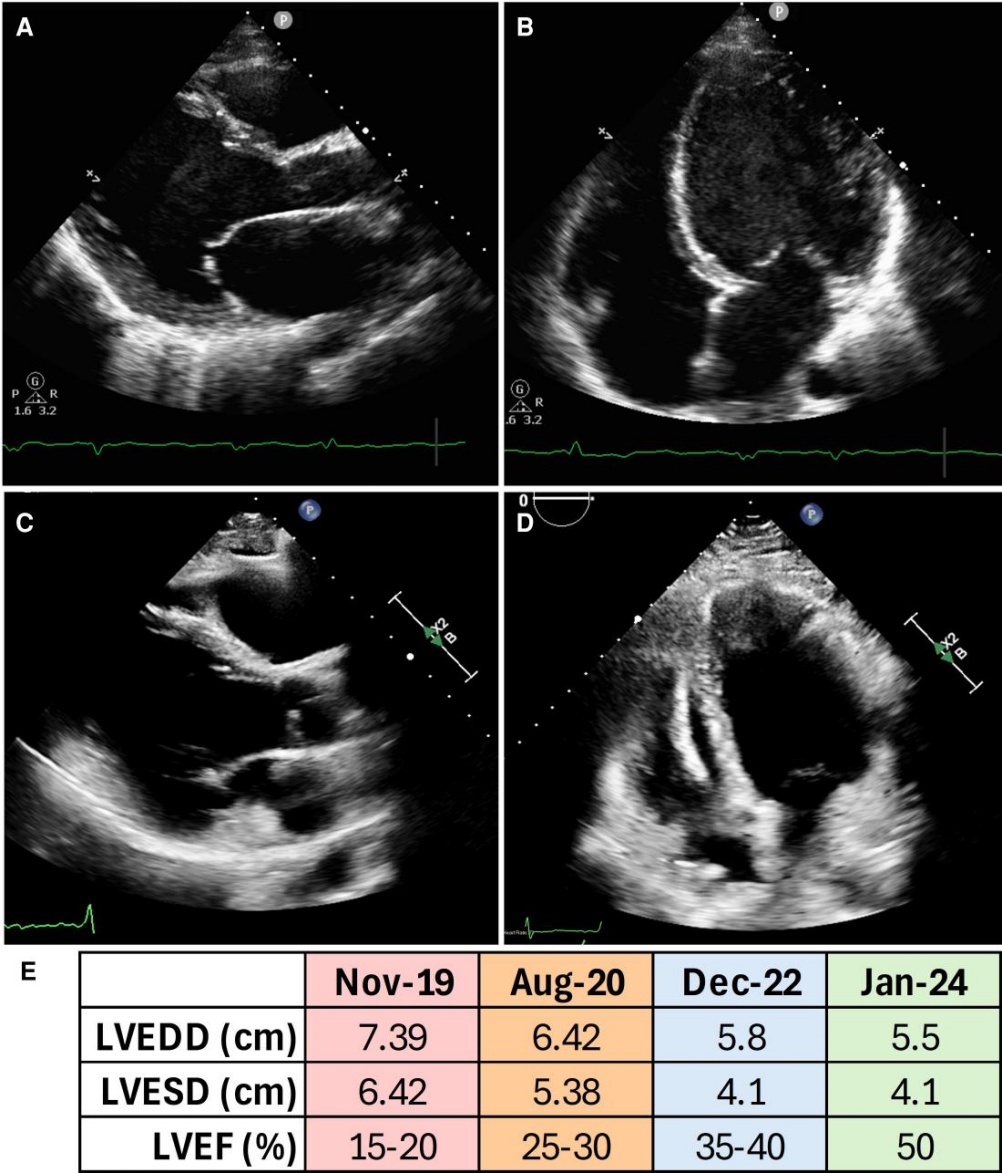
Visual and numeric demonstration of the improvement of the patient’s left ventricular structure and function over time. Parasternal long axis (*A* and *C*) and apical four-chamber (*B* and *D*) views from the patient’s initial and most recent echocardiograms, performed in November 2019 (*A* and *B*) and January 2024 (*C* and *D*), respectively. There is clear visual improvement in left ventricular dilatation. Panel (*E*) presents measurements of the patient’s left ventricular end-diastolic diameter, left ventricular end-systolic diameter, and left ventricular ejection fraction from serial imaging. An improvement of these parameters is observed over time.
